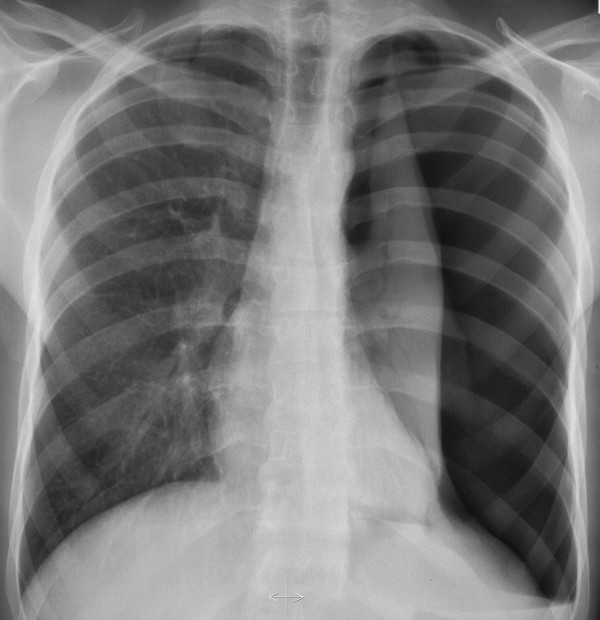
Chest radiography with left lung collapsed and slight mediastinal shift.
